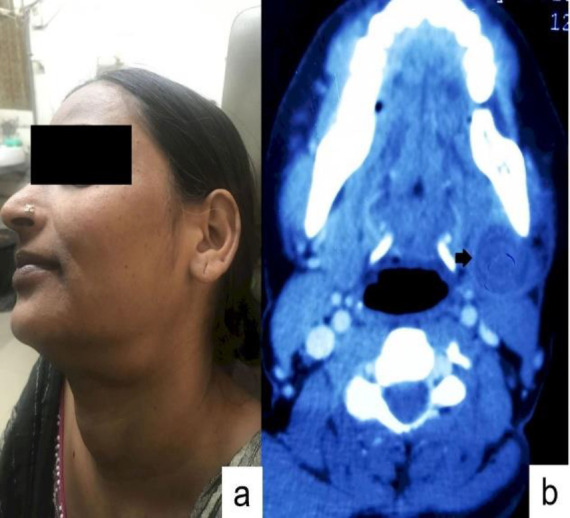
Clinical photograph of the patient showing a submandibular swelling. b: CT scan image [axial cut] delineating the hydatid cyst
